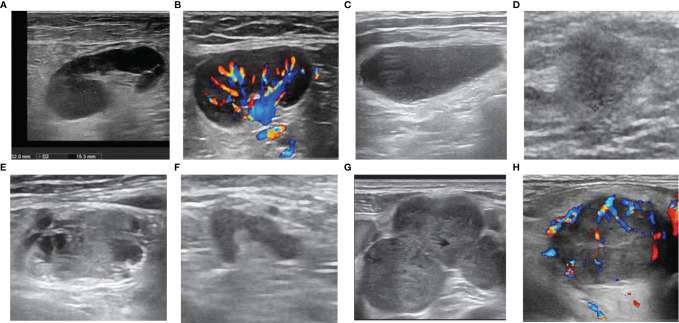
Ultrasonographic features of lymph nodes (LNs). LNs with (A) sharp border, regular margin, hilum, homogeneous cortex, (B) hilar vascularity, (C) absence of hilum, (D) blurred border, (E) calcification and inhomogeneous cortex (necrosis), (F) irregular margin, (G) fusion, (H) peripheral vascularity.
